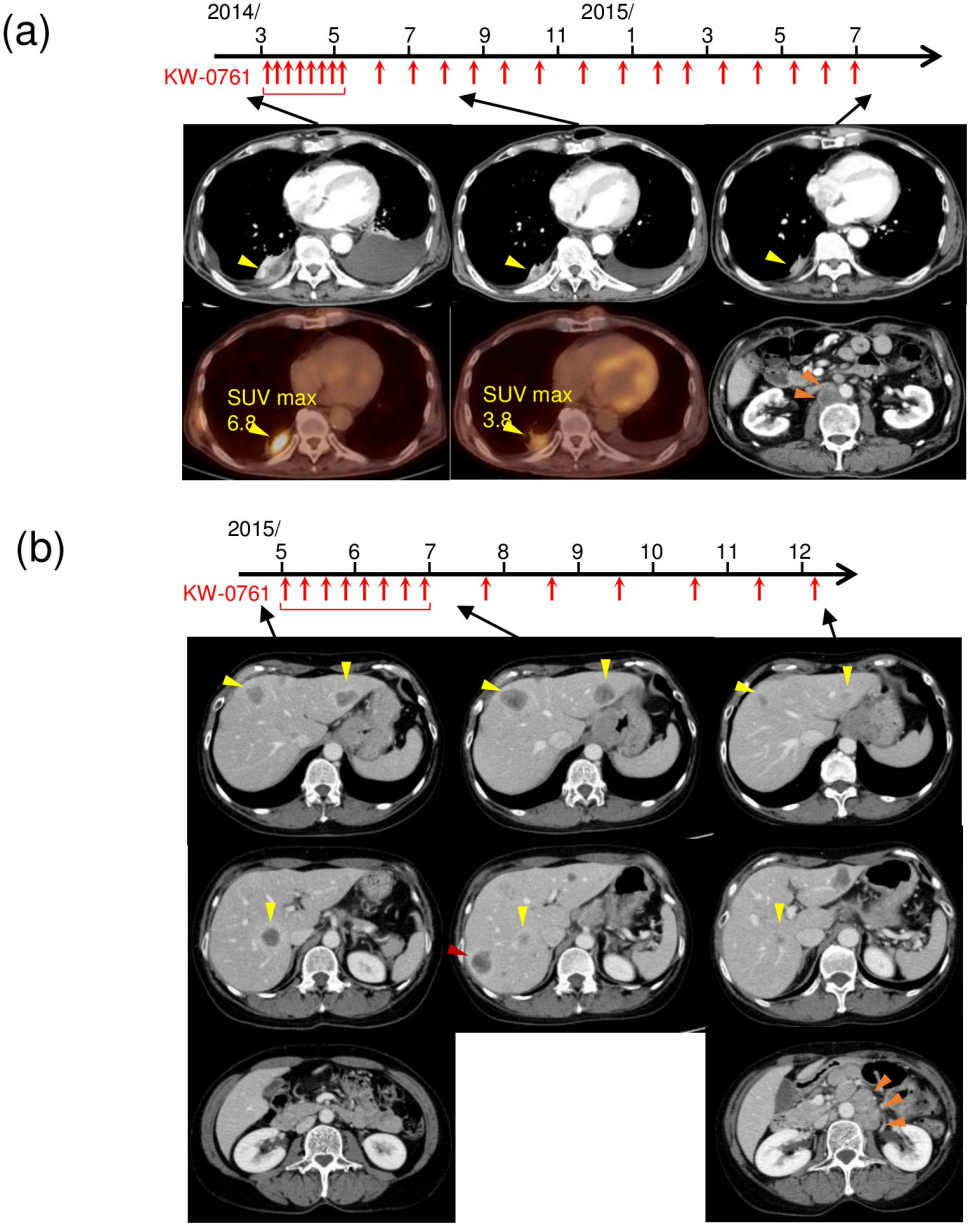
Representative CT imaging in patients with a durable clinical response. (a) Esophageal cancer patient B-09. Pleural metastasis (yellow arrow) was observed on CT and FDG-PET at baseline. The pleural tumor was decreased in size by the KW-0761 treatment, which was also confirmed by a reduction in standardized uptake value-max (SUV-max) on FDG-PET. Although pleural metastasis shrank, abdominal lymph node metastasis (orange arrow) developed after 23 infusions, which was evaluated as a new lesion, leading to the discontinuation of treatment. (b) Esophageal cancer patient B-39. Multiple liver metastases (yellow arrow) were observed on CT at baseline. Disease progression and a new lesion in the liver (red arrow) were confirmed after the first 8 infusions; however, all lesions subsequently decreased in size. The objective feasible response was sustained until 36 weeks after the first treatment when abdominal lymph node metastasis developed (orange arrow).
